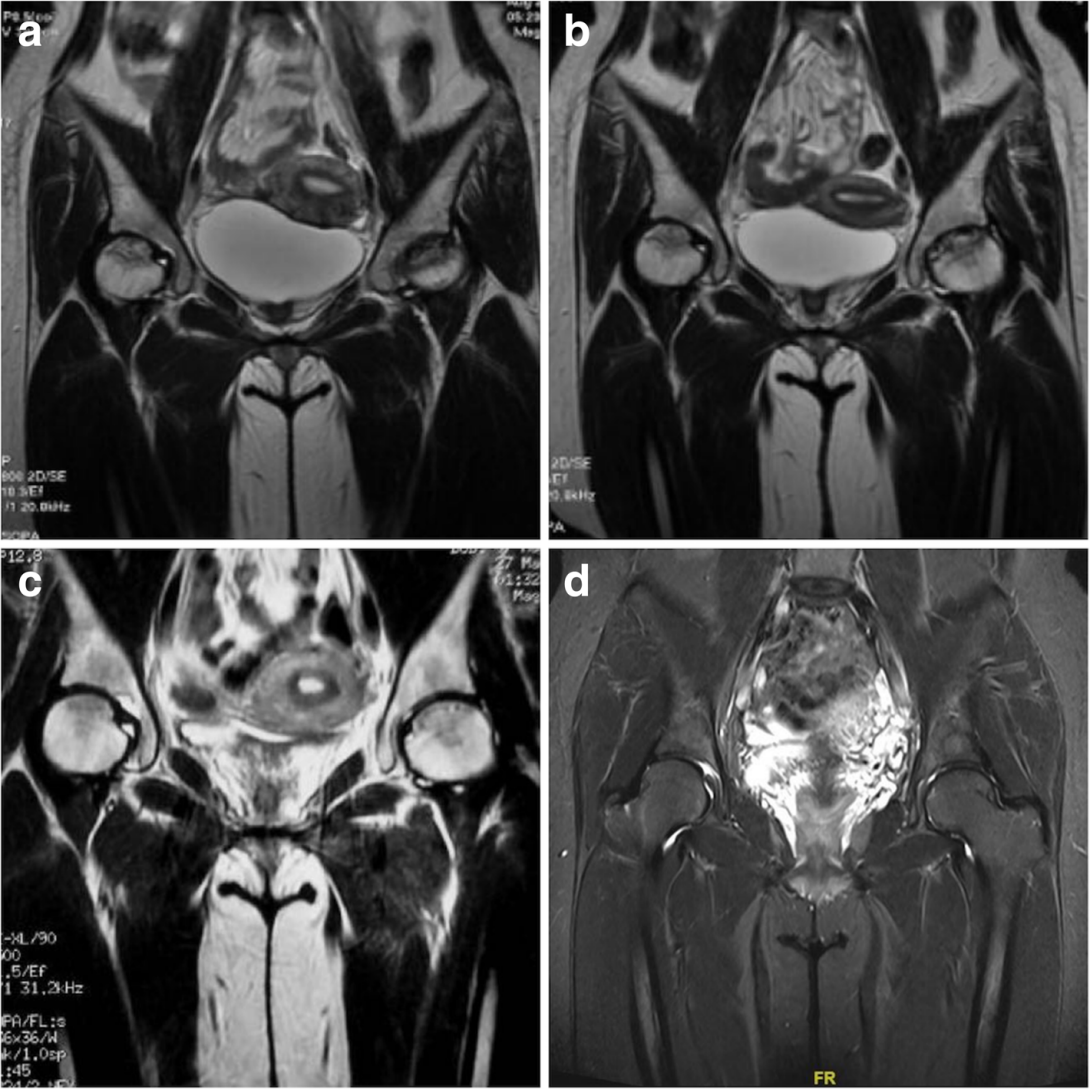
MRI of a young woman with high-dose corticosteroid use: a MRI indicated bilaterally ARCO stage II ONFH before ESWT; b MRI in 3 months after ESWT; c MRI in 5 years after ESWT; d No lesion was observed in MRI at final follow-up. The patient has fully hip function without pain at the final follow-up
